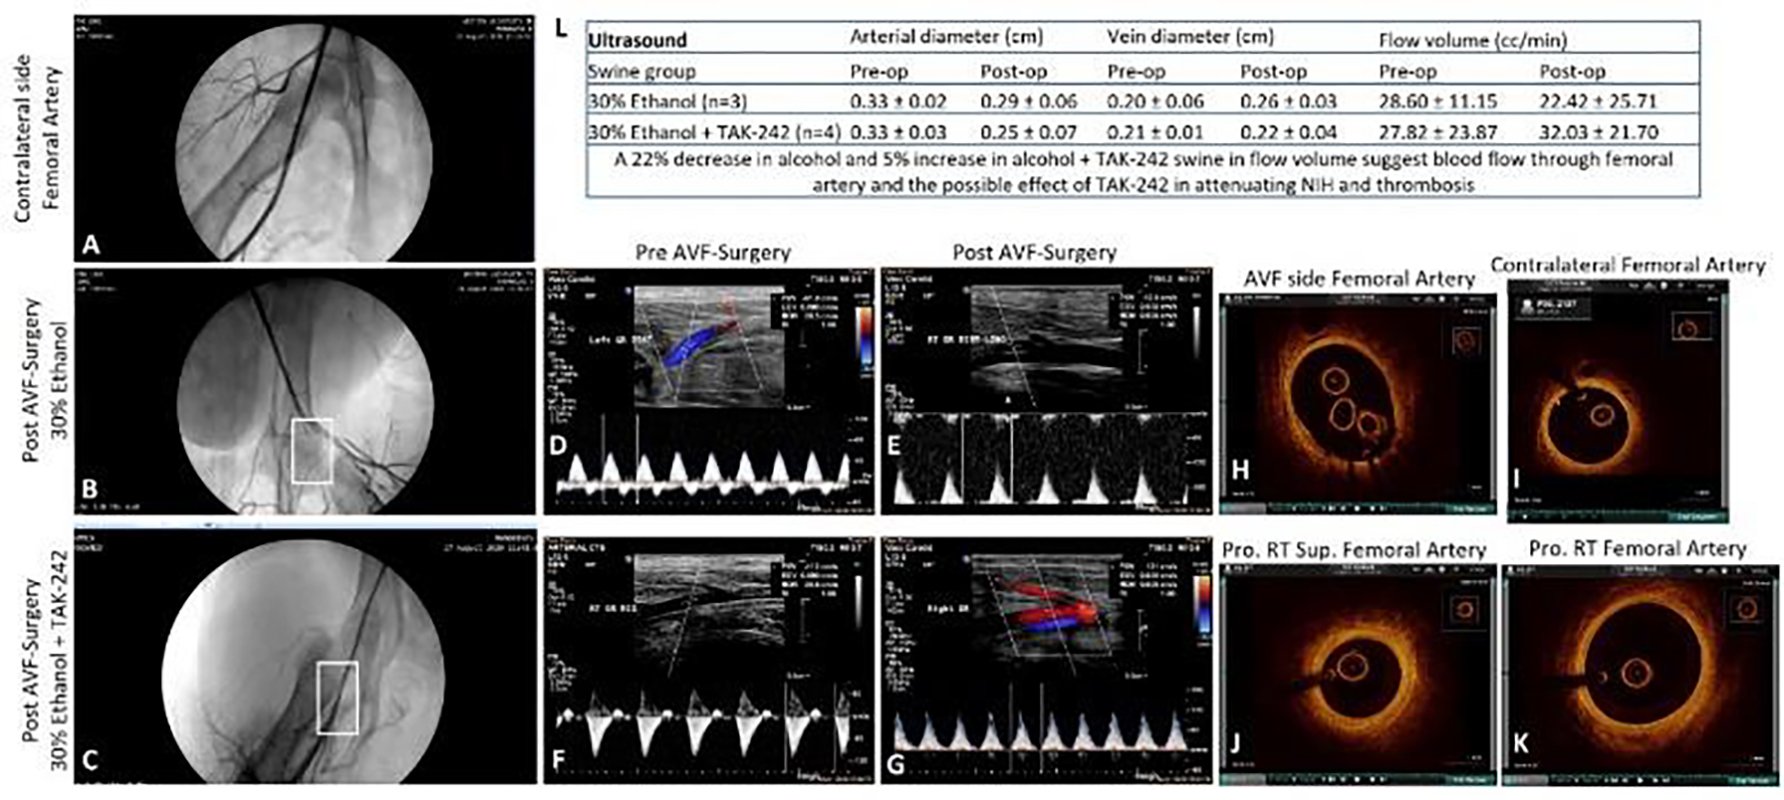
Ultrasound, angiography, and optical coherence tomography. Angiography of the contralateral femoral artery as control and pre-and post-intervention (panels A. B, and C), ultrasound (USG) of femoral artery pre-and post-intervention (panels D-G), and optical coherence tomography (OCT) images of femoral artery pre-and post-intervention (panels H, J, and K), OCT images of the contralateral femoral artery as control (panel I) in ethanol and ethanol + TAK-242 group. Panel L shows the arterial and vein diameter and arterial flow volume before and after the intervention.
